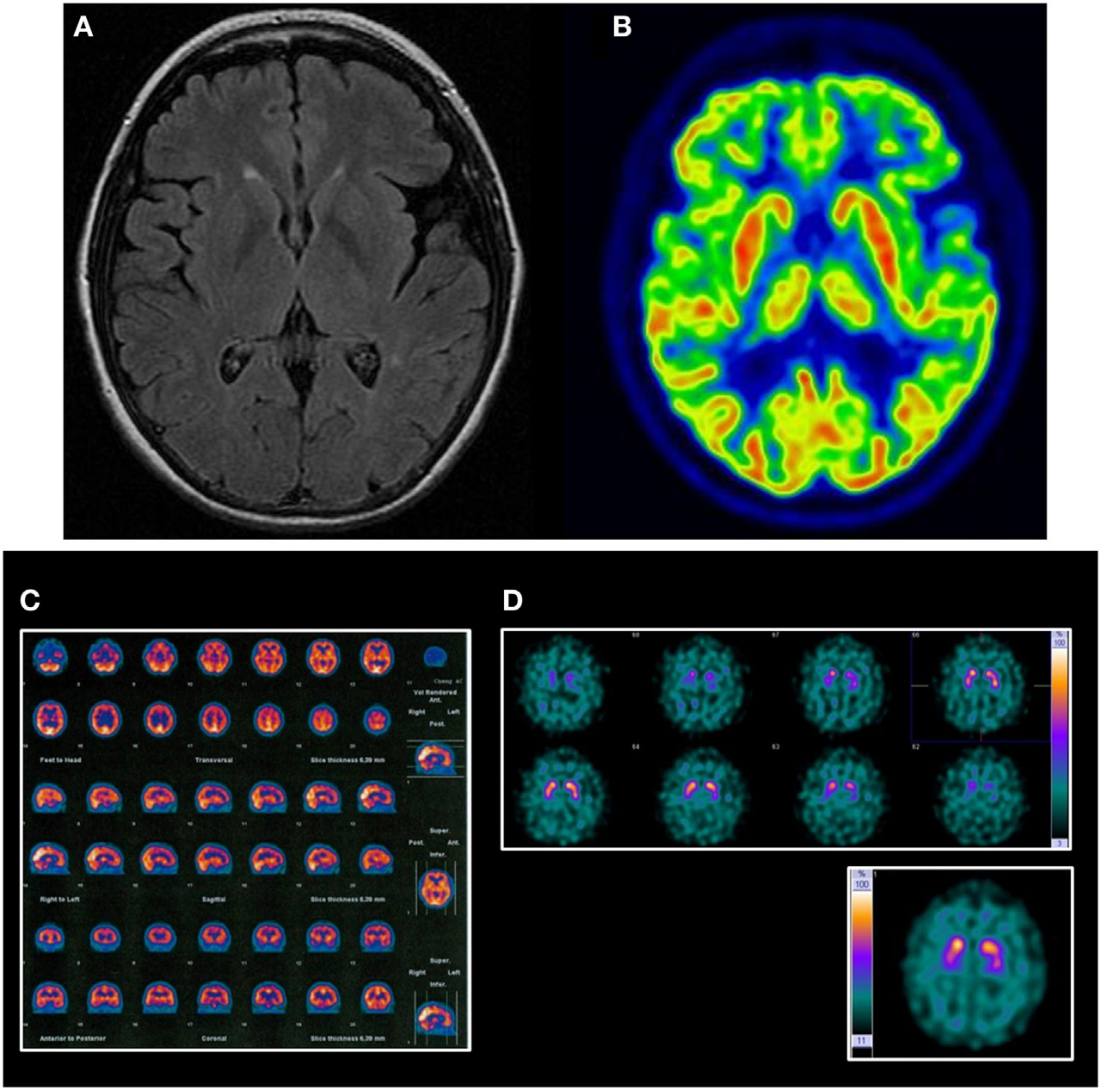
Neuroimaging findings in the reported case. (A) Follow-up MRI mild diffuse atrophy with more intense atrophy on the left frontal and temporal lobes and some small vessel lesions affecting periventricular and semioval white matter. (B) FDG-PET shows mild-to-severe hypometabolism on the left frontotemporal lobe junction and mild hypometabolism on the left fronto-basal, left anterior cingulum regions. (C) HMPAO SPECT shows bilateral but asymmetric hypoperfusion (more on the left side) on frontotemporal lobes. (D) Dopamine transporter-single, photon emission computed tomography was informed of the normal density ofpresynaptic dopaminergic uptakers. Some irregularities in the morphology of basal ganglia and increase of cortical uptake can be noted.
